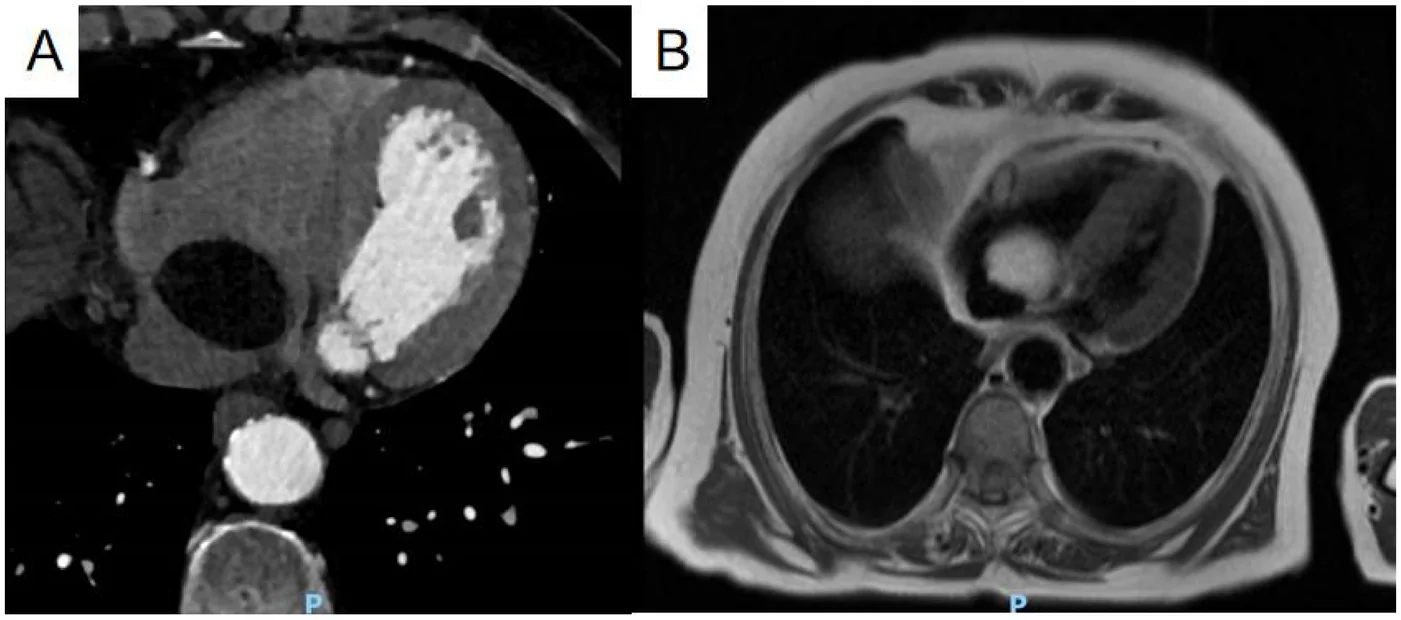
Imaging features of cardiac lipoma. CT imaging of cardiac lipoma shows a hypodense lesion (A), while MRI reveals a fat signal (B).
